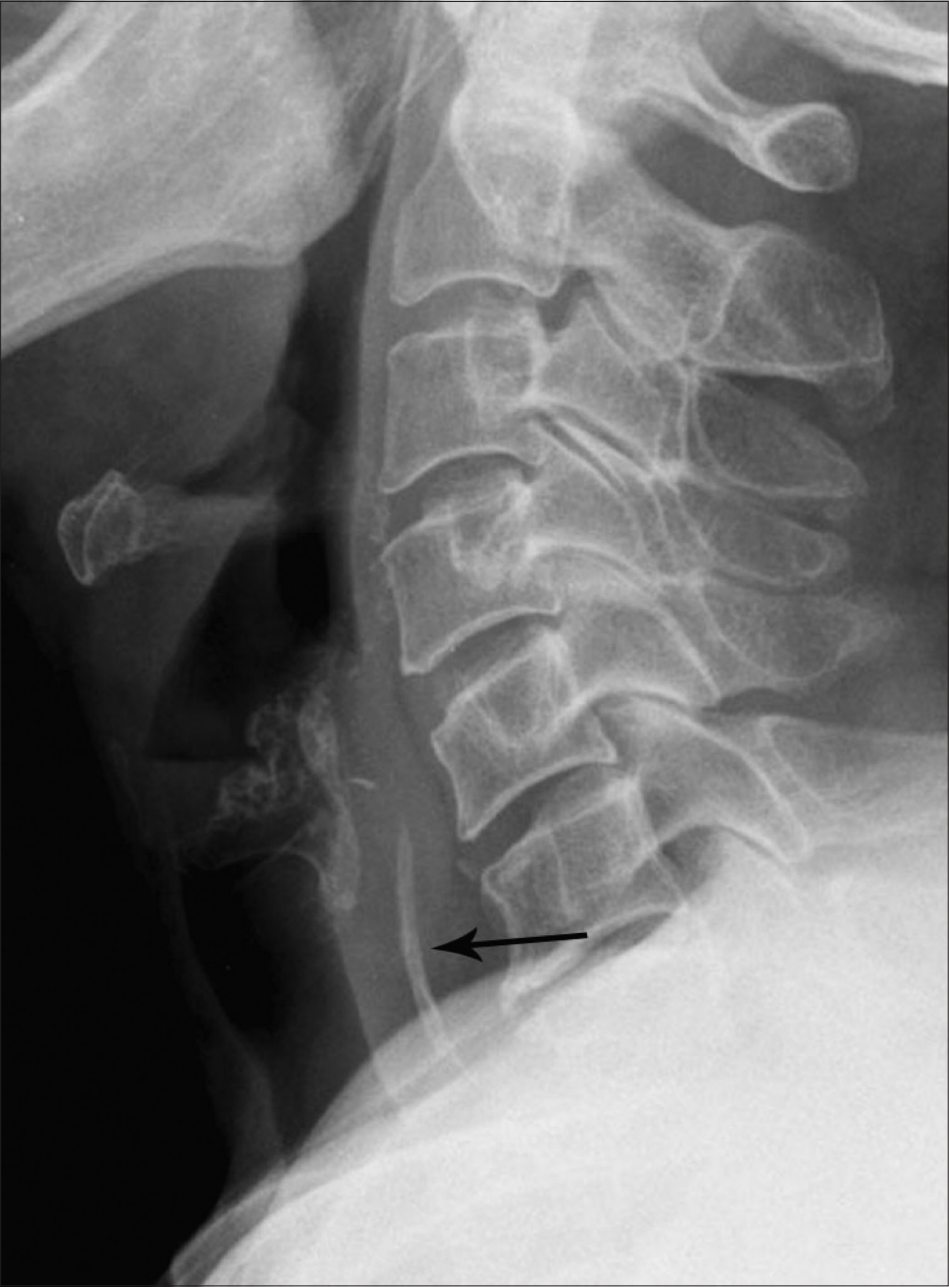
Lateral radiograph of the neck of an elderly patient shows a linear FB within the hypopharynx at the C5-6 level (black arrow). No evidence is seen of any thickening of the prevertebral soft tissues or cervical emphysema
